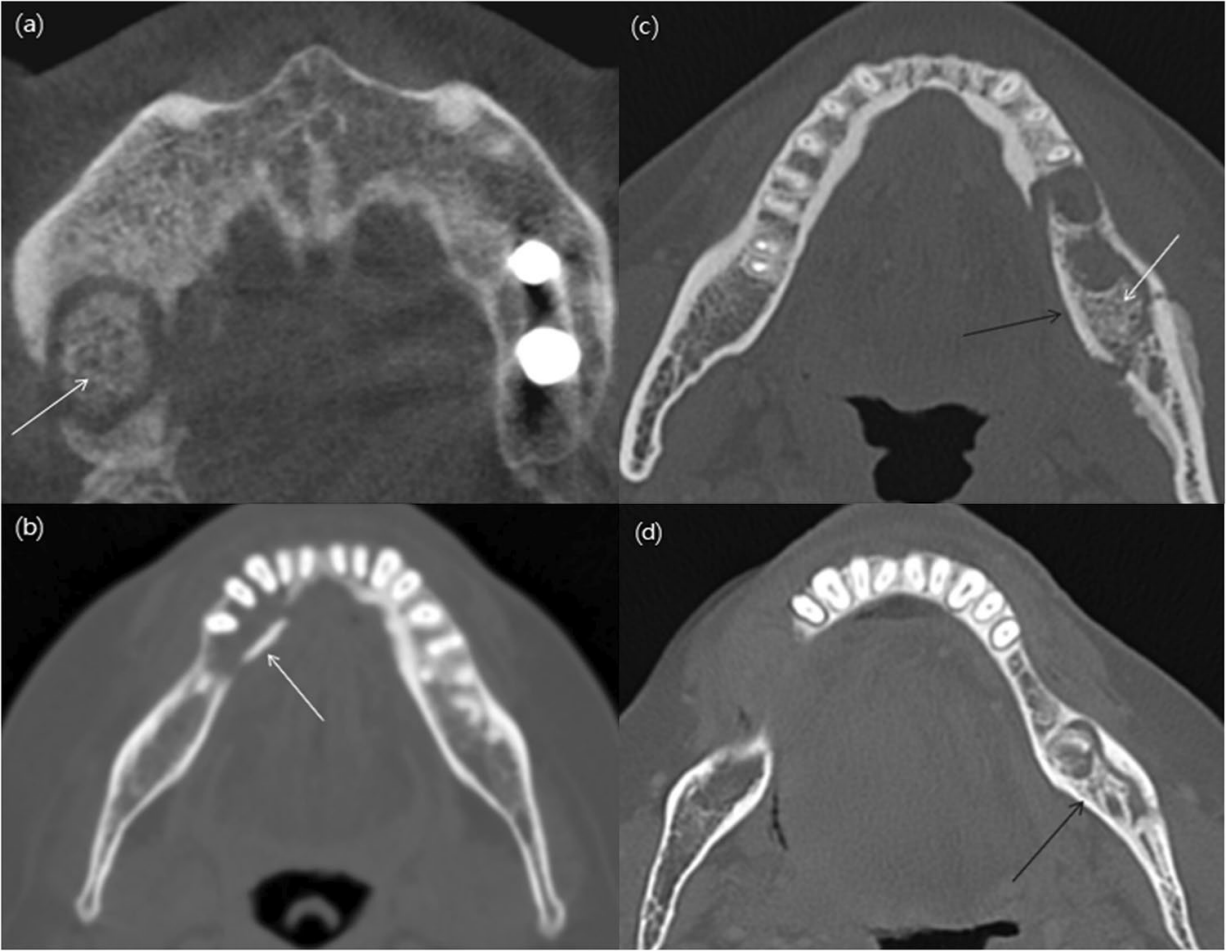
Analysis of hard tissue changes on computed tomography bone window images reveals various types of sequestra formation: (a) trabecular sequestrum; (b) cortical sequestrum; (c) mixed trabecular and cortical sequestrum, which includes both trabecular and cortical bones detached in a block; and (d) incomplete sequestrum, wherein part of the sequestrum is attached to the surrounding bone.
